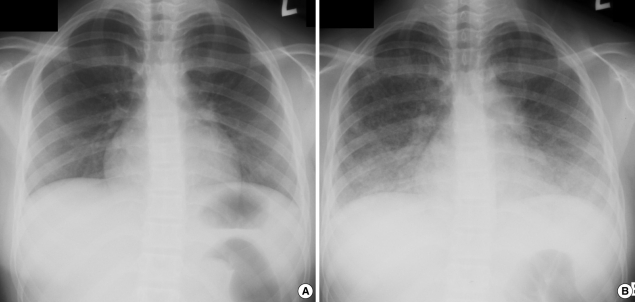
Chest X-ray findings. (A) Mild reticular densities were found in both lung fields (June 16th, 2006), and (B) increased reticular densities were mainly observed in both lower lung fields. Small nodular densities were newly developed, but neither cardiomegaly nor pleural exudates were observed. Perihilar lymph node enlargement was suspected (June 25th, 2006, postmortem).
